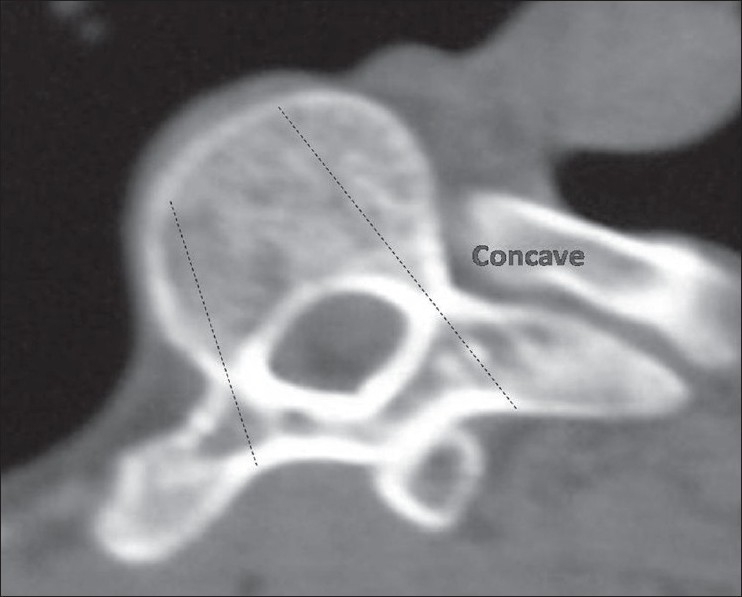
Axial CT image depicting depath to anterior cortex/chord length-Concave and convex sides
